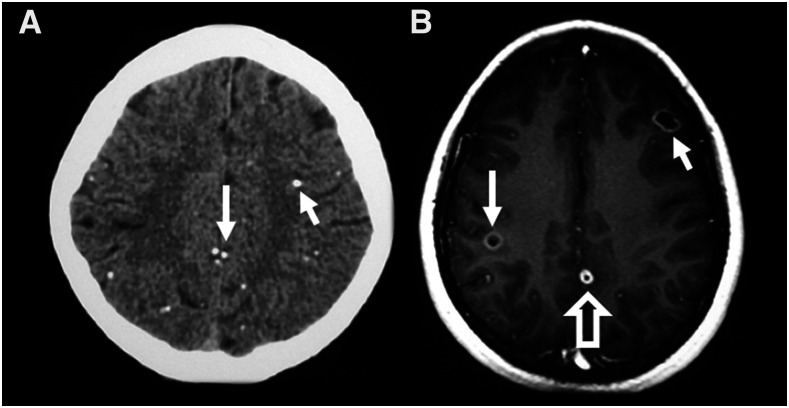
Burden of disease. An increased number of lesions may be associated with an increased likelihood for treatment-resistant seizures in subjects with NCC. (A) Axial computed tomography (CT) scan of a patient with NCC who had multiple calcified lesions. Representative calcifications on this image are indicated by solid arrows. (B) Axial T1 MRI scan demonstrating multiple degenerating lesions. The image shows two lesions in the colloidal stage of degeneration that are indicated with solid arrows; a lesion in the nodular stage is indicated with a hollow arrow.
